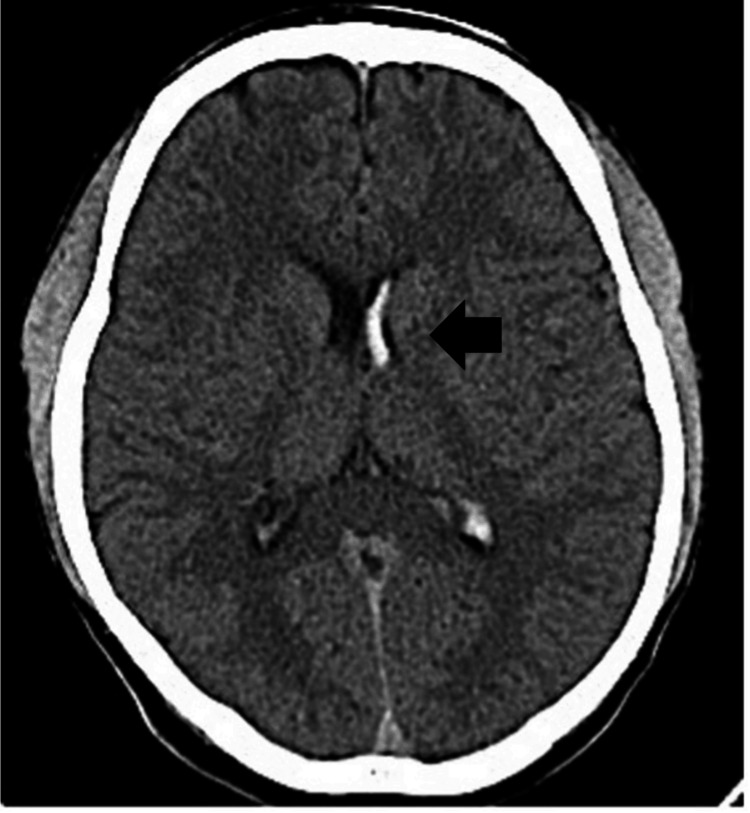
Axial image of a noncontrast computed tomography scan at the onset showing a small intraventricular hemorrhage (black arrow) at the left lateral ventricle.
